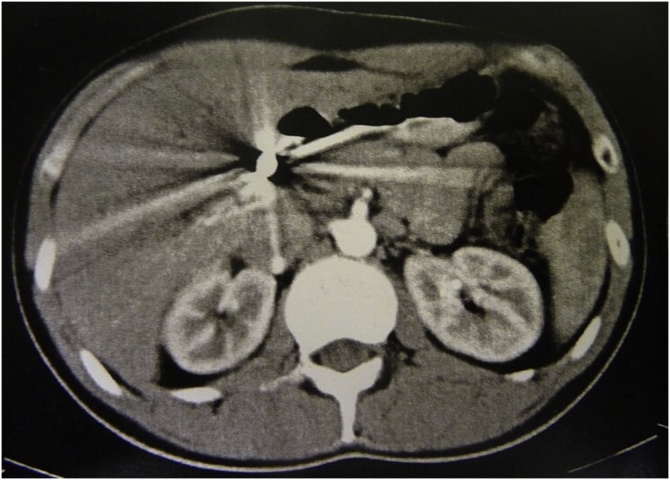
Computed tomography (CT) with visualization of metallic fragment near at biliary tract and hepatic hilum.
